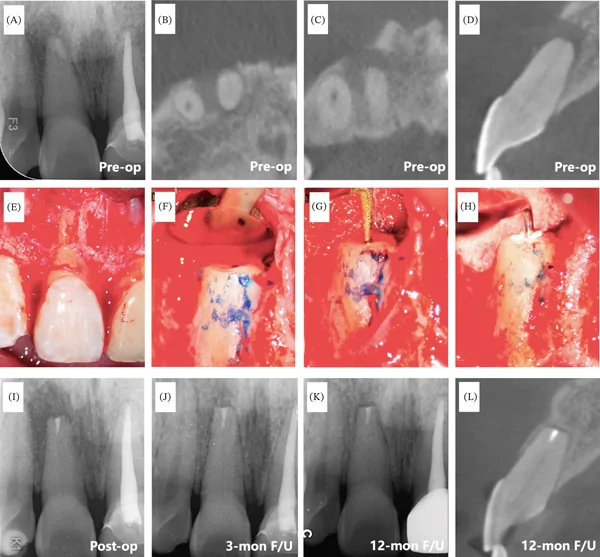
Preoperative, intraoperative, and postoperative imaging of Case 1 (Tooth #8). (A) Preoperative PA demonstrating PCO with an associated periapical radiolucency of Tooth #8. (B–D) Preoperative CBCT images revealing an indistinct root canal, a large periapical radiolucent lesion, and complete resorption of the labial cortical plate of Tooth #8. (E) Intraoperative photograph following flap reflection showing a labial bone dehiscence extending to the root apex. (F) Resection of the apical 3 mm. (G) Ultrasonic retrograde preparation to a depth of 3 mm. (H) Root‐end filling with iRoot BP Plus. (I) Immediate postoperative PA of Tooth #8. (J) Three‐month follow‐up PA showing significant resolution of the periapical lesion. (K,L) Twelve‐month follow‐up PA and CBCT image demonstrating complete periapical healing and regeneration of the labial cortical plate. CBCT, cone beam computed tomography; PA, periapical radiograph.
